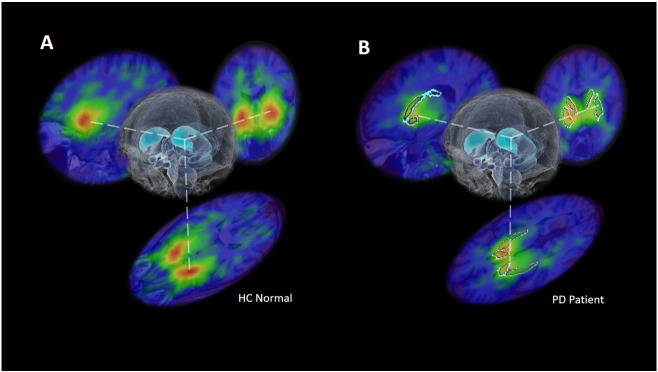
Examples of transaxial, coronal and sagittal slices through the DaT SPECT images for a HC subject (A) and a PD subject (B), also showing segmentation for caudate and putamen (B).
